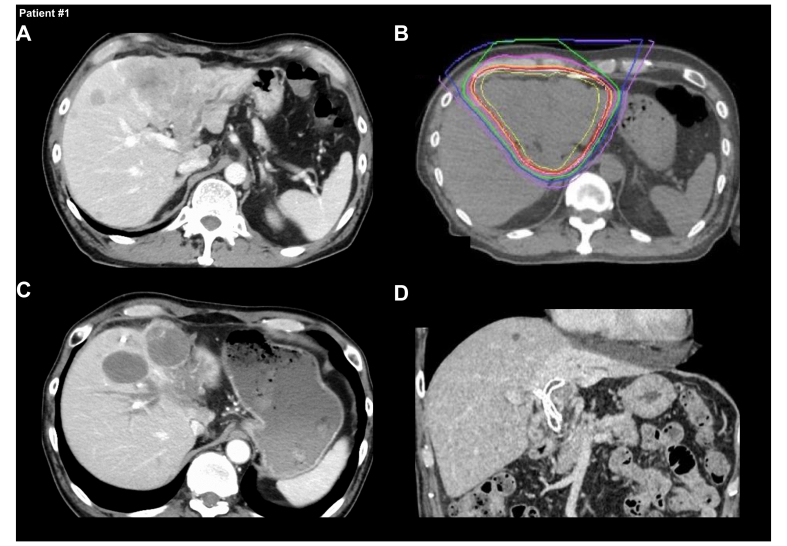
CT imaging of the case which developed intrahepatic bile duct dilatation during long-term observation. (A) CT scan imaging of baseline. (B) Dose distribution of C-ion RT superimposed on contrast-enhanced computed tomography (B). (C) Bile duct dilatation development. (D) After stenting (metallic stent) in the bile duct. C-ion RT, carbon-ion radiotherapy; CT, computed tomography.
